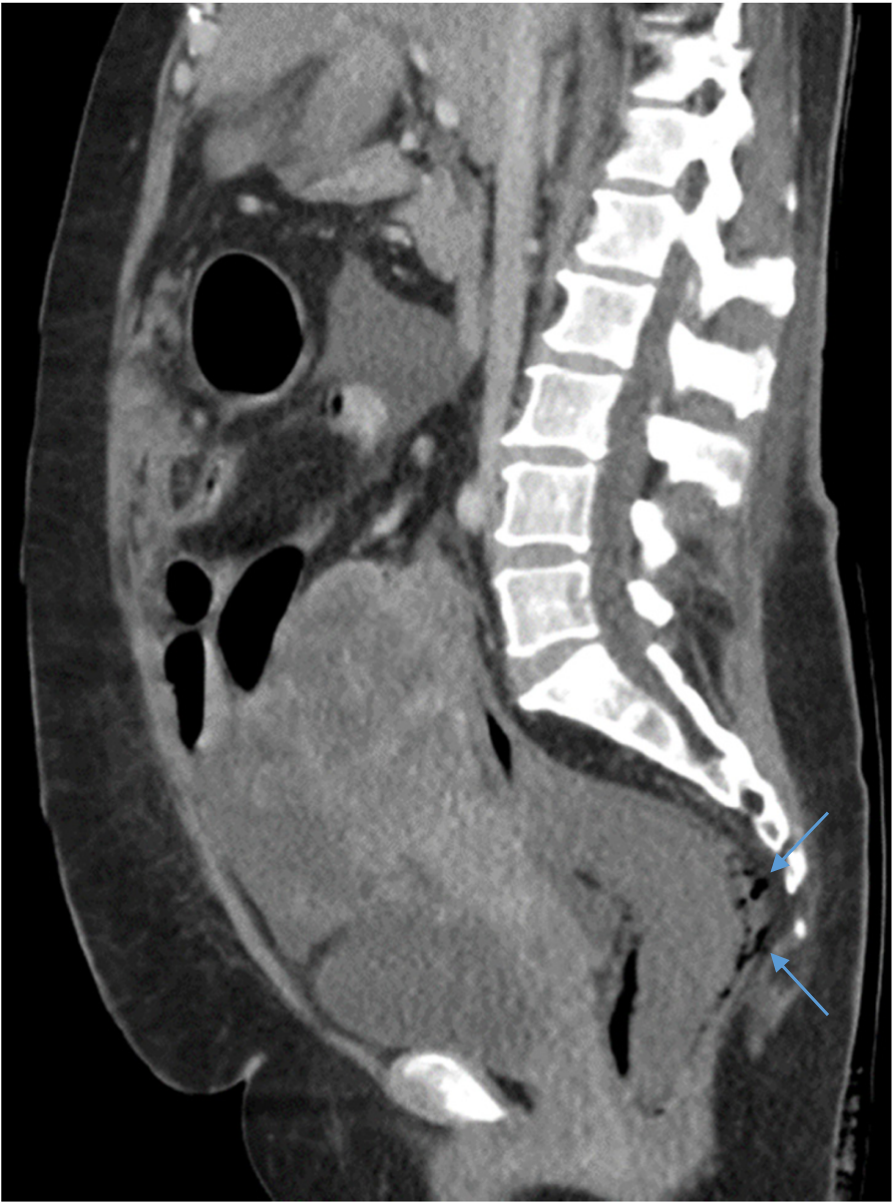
Rectal perforation. Free air is present outside the rectum as indicated by *arrows* on sagittal section of computed tomography scan of the pelvis
